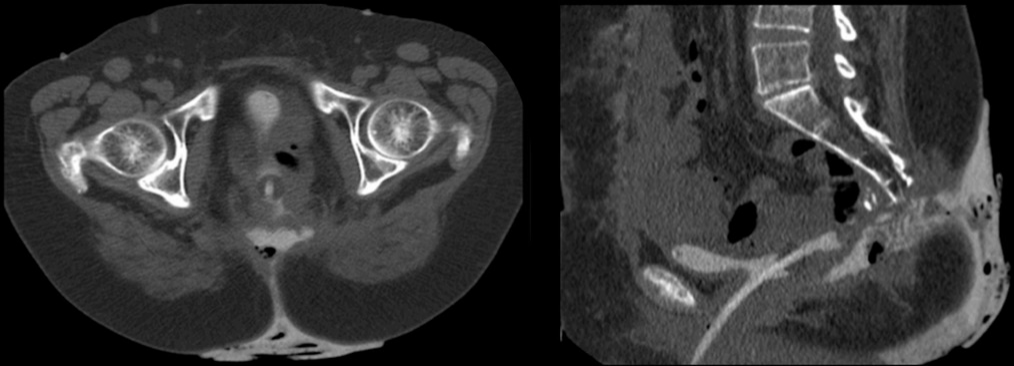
Axial (A) and sagittal (B) CT pelvis following contrast injection via a Foley catheter shows the posteriorly sited urinary bladder wall defect.
